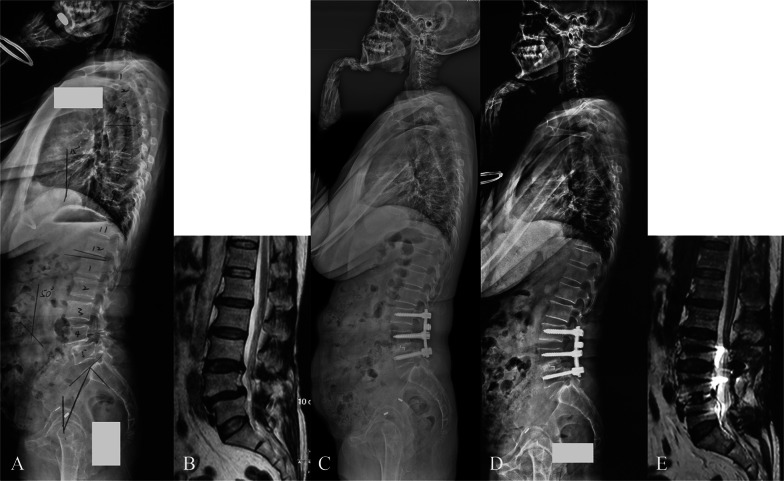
A case with matched Roussouly type. A 60-year-old female with lumbar spinal stenosis and isthmic spondylolisthesis at L3–4 and L4–5, the theoretical Roussouly shape was type 3 based on the PI of 57° (A, B). She underwent pedicle screw fixation from L3 to L5, and posterolateral inter-transverse process fusion at L3–4 and TLIF at L4–5. The upright lateral radiograph showed that PT was 20°, SS was 37°, LL was 49°, DL was 35°, LDI was 71%, lumbar apex was L4, and inflexion point was L1 (indicating the type 3 shape and a matched status, C). At 5-year follow-up, she reported being well. The upright lateral radiograph and MRI showed no signs of ASD (D, E). *PI* pelvic incidence, *PT* pelvic tilt, *SS* sacral slope, *LL* lumbar lordosis, *DL* distal lordosis, *LDI* lordosis distribution index, *TLIF* transforaminal lumbar interbody fusion, *MRI* magnetic resonance imaging, *ASD* adjacent segment disease
